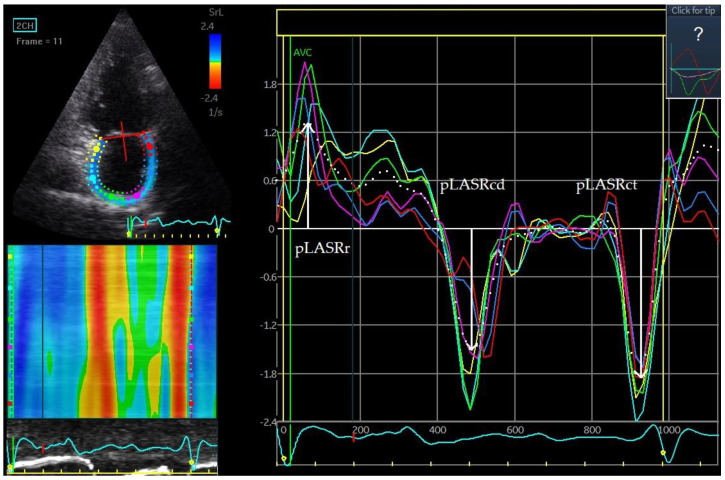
Left atrial strain rate. pLASRr 1.3 s^−1^ peak left atrial strain rate measured during the reservoir phase. pLASRcd −1.5 s^−1^, peak left atrial strain rate measured during the conduit phase. pLASRct −1.9 s^−1^, peak left atrial strain rate measured during the contractile phase. The measurements are the average of the 6 assessed left atrial wall segments. The measurement was made in the apical two-chamber projection during sinus rhythm.
